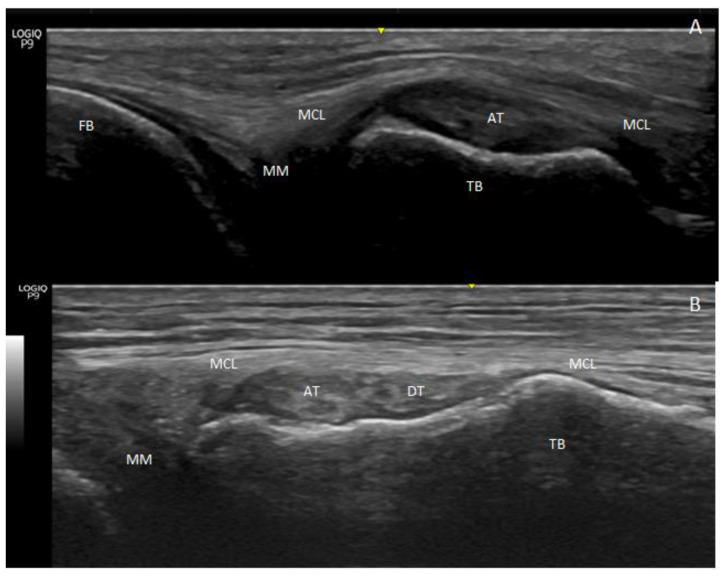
Image of the medial side of the knee. (A) An ultrasound image with the prove at the long axis: the anterior tendon (AT) of the semimembranosus muscle is seen as a round hyperechogenic image covered by the medial collateral ligament (MCL) in a special concavity that the tibia bone (TB) forms at the point of insertion. (B) Ultrasound vision of the two round hyperechogenic structures compatibles with the anterior tendon (AT) and direct tendon (DT) of the semimembranosus. Medial meniscus—(MM) and femur bone—(FB).
